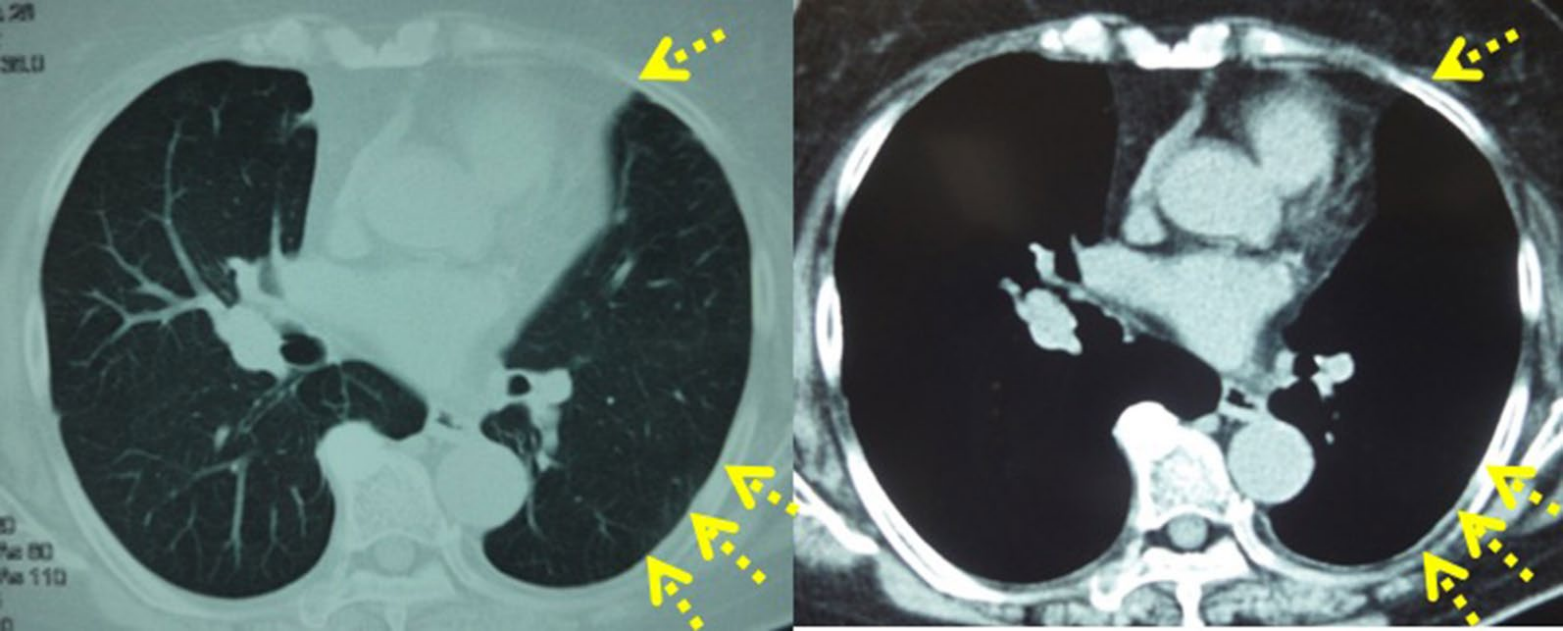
Complete recovery from pleural metastasis could be confirmed by a chest CT scan performed 1 year after endocrine therapy was started. Arrows (→) show disappearance of pleural metastasis and pleural effusion
